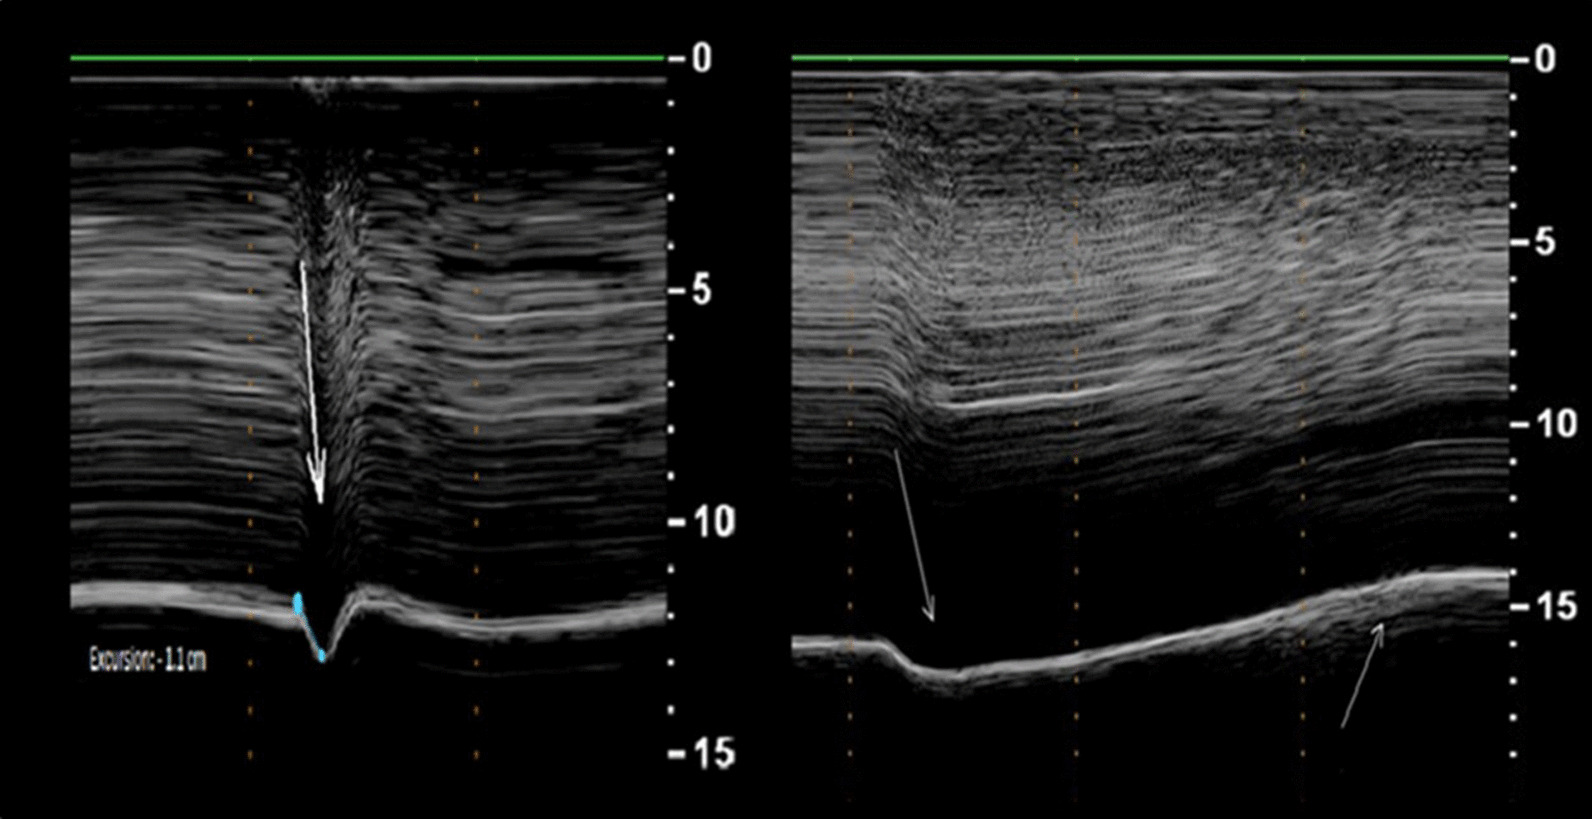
M-mode ultrasonography in a representative patient with hemidiaphragm paralysis recorded during a sniff (left panel) and during deep breathing (right panel). During a sniff (left panel), the paralyzed hemidiaphragm show a paradoxical motion (arrow). During deep breathing (right panel), the hemidiaphragm displays a biphasic movement characterized by an initial paradoxical movement (left arrow) and a terminal caudal displacement (right arrow). (Reproduced with permission from John Wiley and Sons: Boussuges et al. Clin Physiol Funct Imaging 2019;39(2):143–9)
